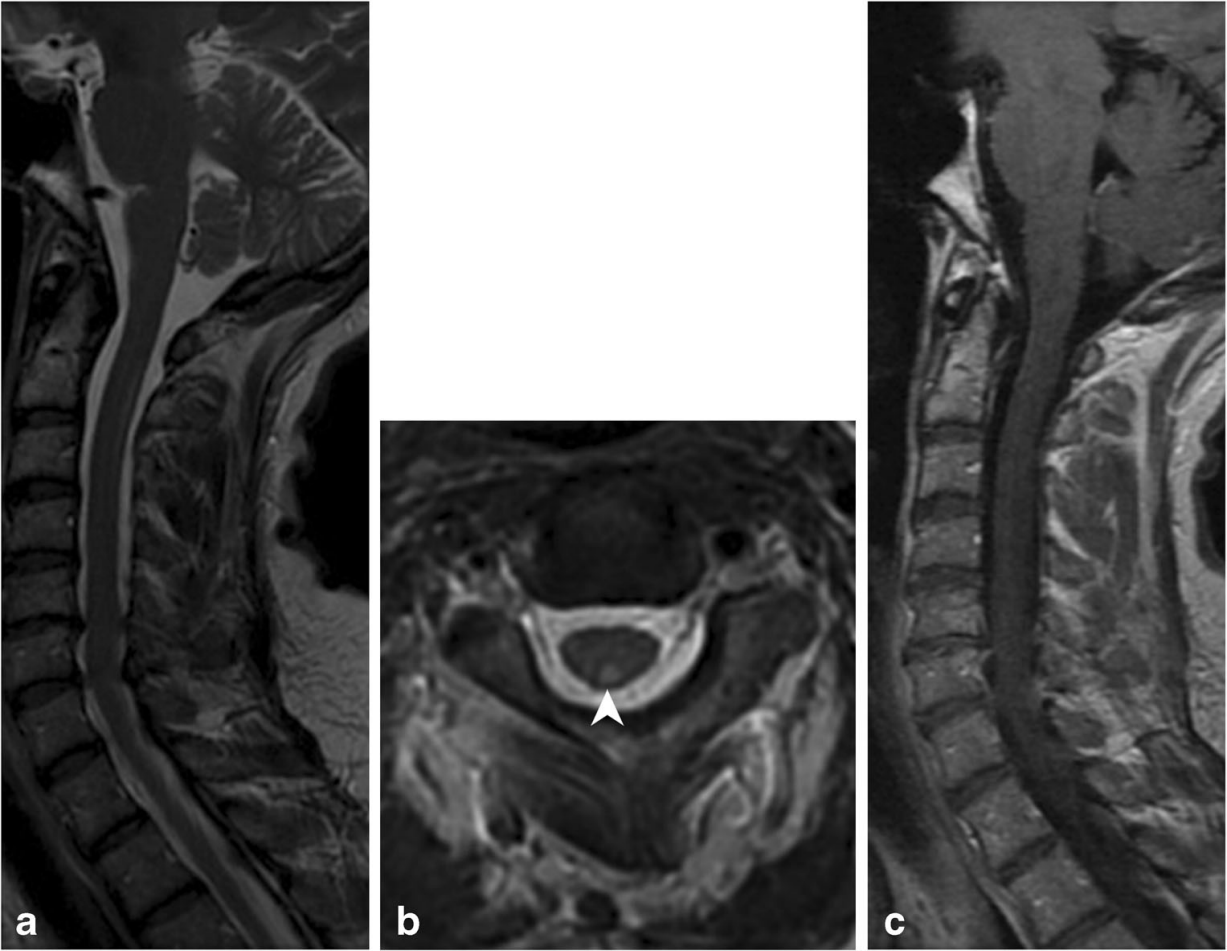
HIV myelitis. A 54-year-old female with a history of HIV presents with peripheral neuropathy. a Sagittal T2-weighted image demonstrates a long segment of hyperintensity in the dorsal cord. b Axial T2-weighted image shows a well-defined dorsal cord lesion (arrowhead) with normal signal within the remainder of the cord. c Sagittal T1 post- contrast image shows no enhancement
